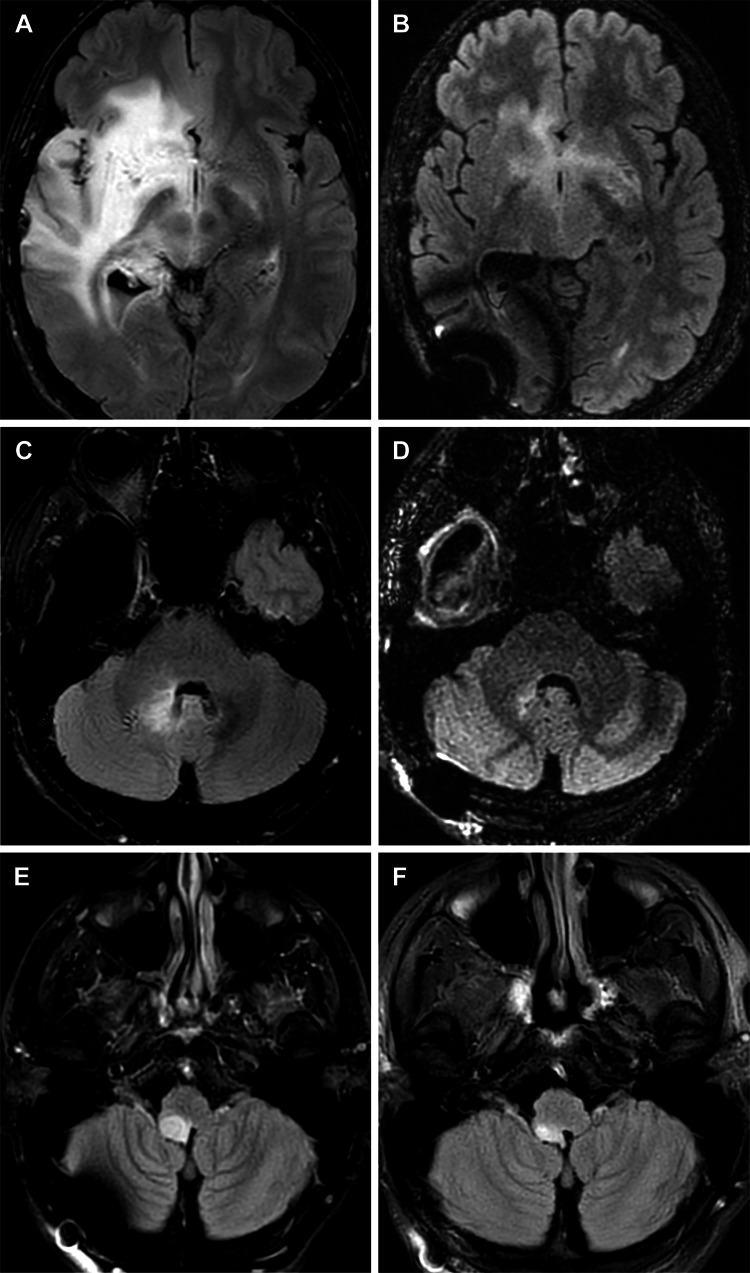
MRIs for patient 1 Axial magnetic resonance images (MRIs) for patient 1, who underwent repeat surgical resection, show that afterward the patient had continued progression of the tumor within the right insula, thalamus, basal ganglia, basal forebrain (A), and cerebellomedullary region (C). After initiation of ketogenic metabolic therapy, tumor-treating fields therapy, and temozolomide therapy, imaging shows near-complete resolution of the diffuse multifocal fluid-attenuated inversion recovery anomaly in the right insula, thalamus, basal ganglia, basal forebrain (B), and cerebellomedullary region (D). After a period of time during which the patient did not achieve ketosis, subsequent imaging showed progression of the tumor within the right medial temporal lobe and right medulla (E). Metabolic genomic profiling identified the cause of the patient’s inability to achieve ketosis, and he reinitiated ketogenic metabolic therapy. Because of its severity, the cerebellomedullary lesion was treated with Zap-X stereotactic radiosurgery. Follow-up imaging showed that the size of the lesion was greatly reduced (F). Used with permission from Barrow Neurological Institute, Phoenix, Arizona.
